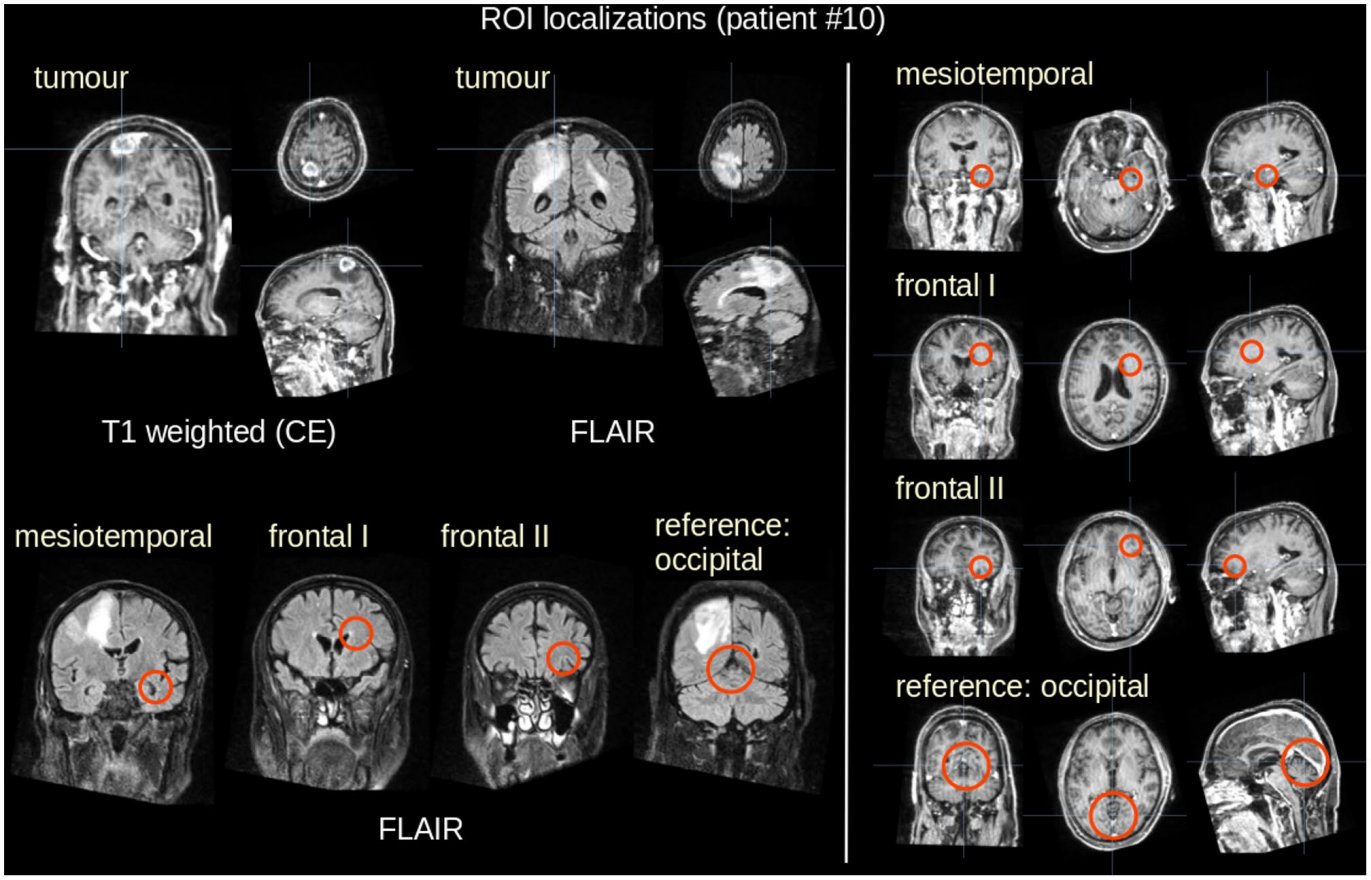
Example of 3D visualization (patient #10, 70  years, male). Left panel: Tumor localization in T1w (contrast-enhanced – CE) and in FLAIR data in coronar, axial, and sagittal representation (upper panel), and mesiotemporal (hippocampal) ROI localization (size 30 mm, lower panel). Right panel: ROI localizations in frontal areas (size 30 mm) and occipital (size 50 mm).
